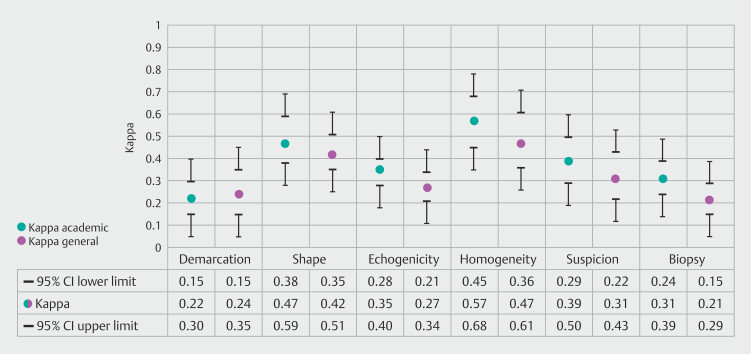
Kappa values per LN characteristic of endosonographers working in academic and general hospitals. CI, confidence interval.
